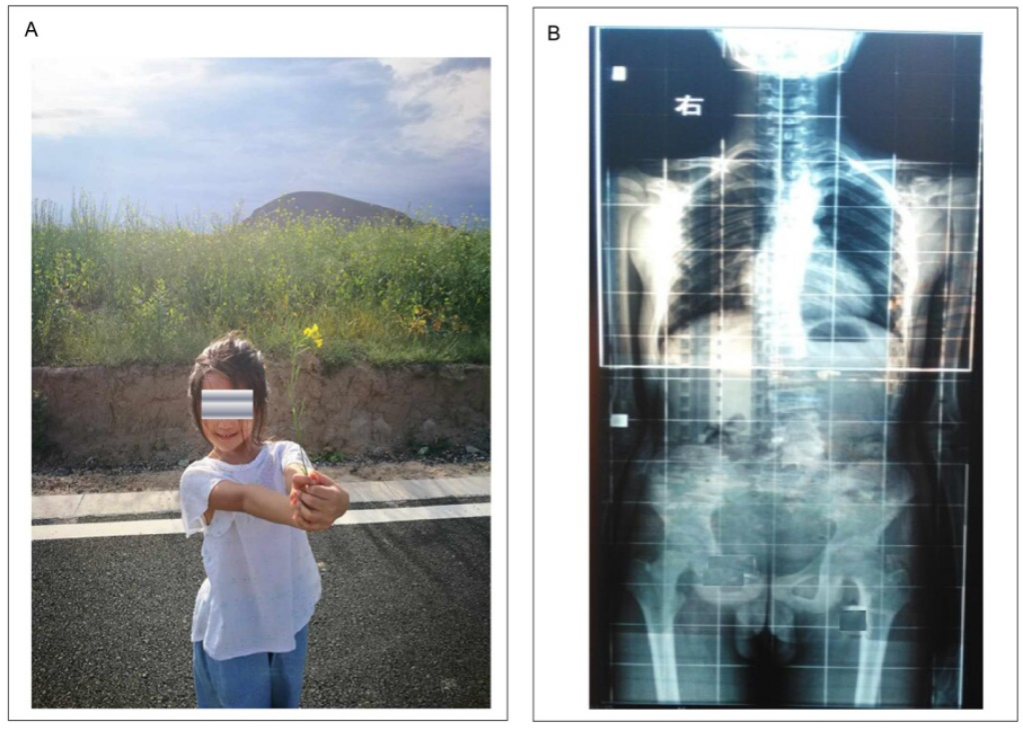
Left: A young female from China with AIS. She was diagnosed AIS aged 20 with a Cobb angle of 30 degrees. Unawareness of the truth on AIS and being afraid of negative effects on her daily life, her parents had to undergo surgery as suggested by a spinal surgeon. Right: Typical whole-spine radiograph of a male with spinal scoliosis. Note AP projection with loose collimation and wide radiation field. The patient and his parents were not aware of radiation exposure.
